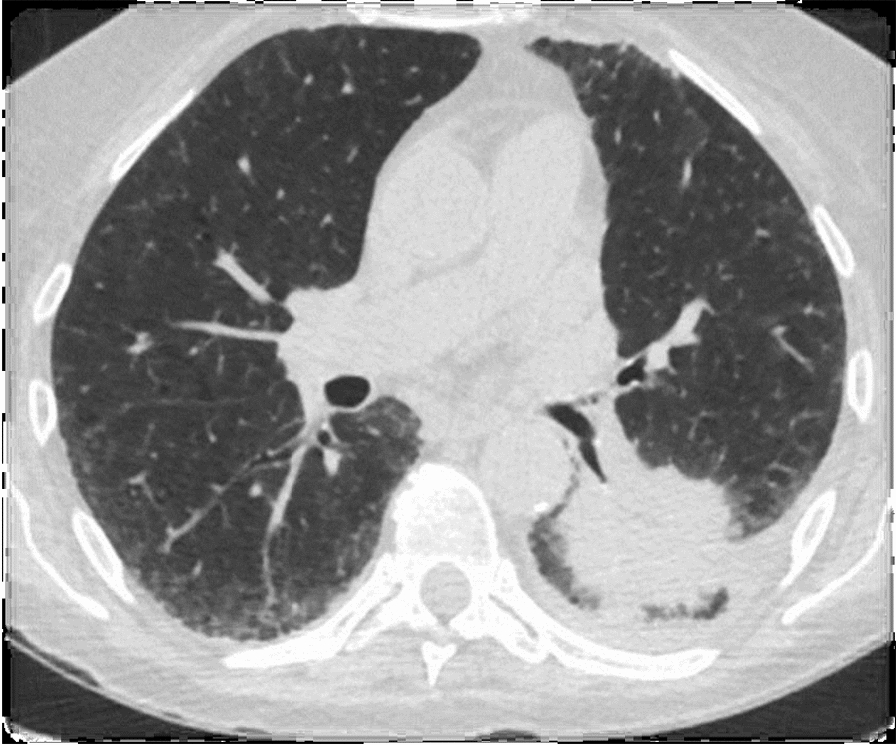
Malignant lung tumor in a patient with pulmonary fibrosis observed on CT scan
